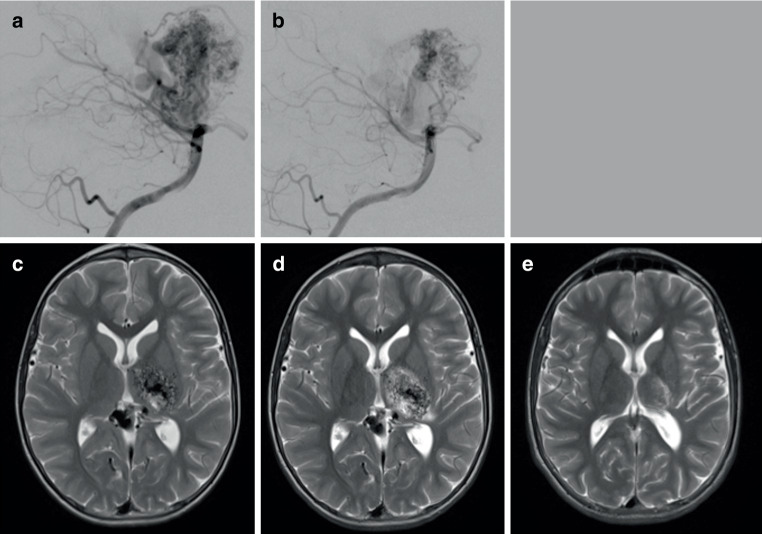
The angiographic appearances pre-embolization (a) and post-multiple embolization sessions including those performed under asystole demonstrated a significant reduction in the volume of the nidus (b). An axial T2-weighted magnetic resonance imaging (MRI) post-embolization demonstrated continued shunting but no major infarction (c). Gamma knife radiosurgery was performed to complete the treatment, which caused some perinidal edema. (d) At year 4 post-radiosurgery there was complete obliteration of the AVM (e)
